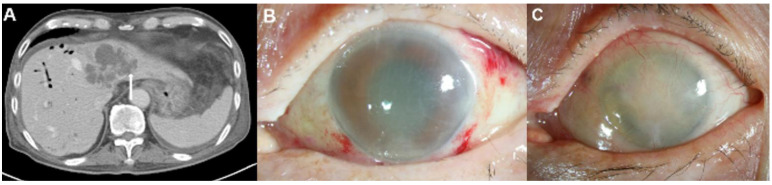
Representative images of an 87-year-old man with diabetes mellitus who presented endogenous endophthalmitis due to *Klebsiella pneumoniae*-related pyogenic liver abscess. (A) This patient had biliary cirrhosis due to recurrent pyogenic cholangitis and underwent contrast computed tomography (CT) examination. The CT image showed a 6.8 cm septated cystic lesion, representing a *Klebsiella* hepatic abscess, at liver segments II and IV. The CT image also showed the hepatic abscess with invasion from the adjacent hepatic vein (arrow). (B) Severe corneal edema and anterior chamber haziness were noted during an initial slit-lamp examination without history of previous ocular surgery. (C) Despite aggressive treatment, his eye developed phthisis.
